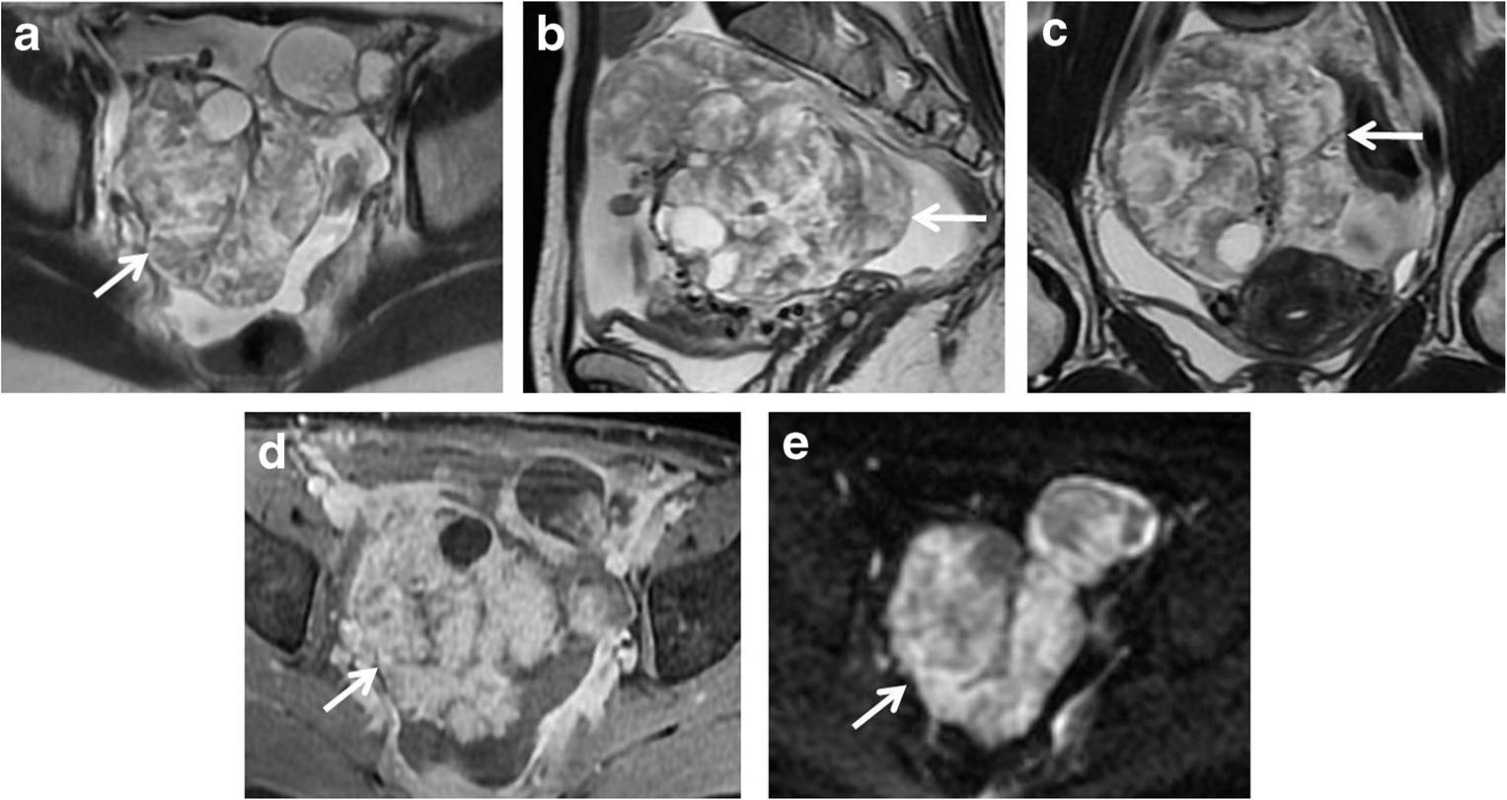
Serous papillary carcinoma in a 48-year-old woman. (a) Axial, (b) sagittal and (c) coronal T2-weighted image show a cystic and solid mass (*white arrows*). (d) Axial contrast-enhanced fat-suppressed T1-weighted image shows the enhancing exophytic papillary projections of the tumour (*white arrow*). (e) Axial DW image (*b* = 800 s/mm^2^) demonstrates increased signal of the lesion (*white arrow*), indicating hypercellularity
